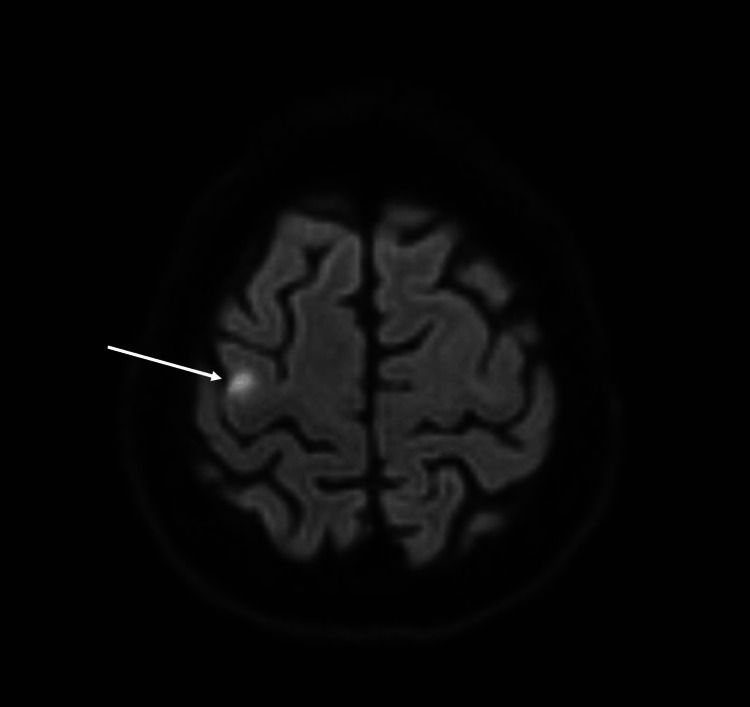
Magnetic resonance imaging diffusion-weighted imaging sequence of the brain showing an acute infarction involving the right precentral gyrus.
